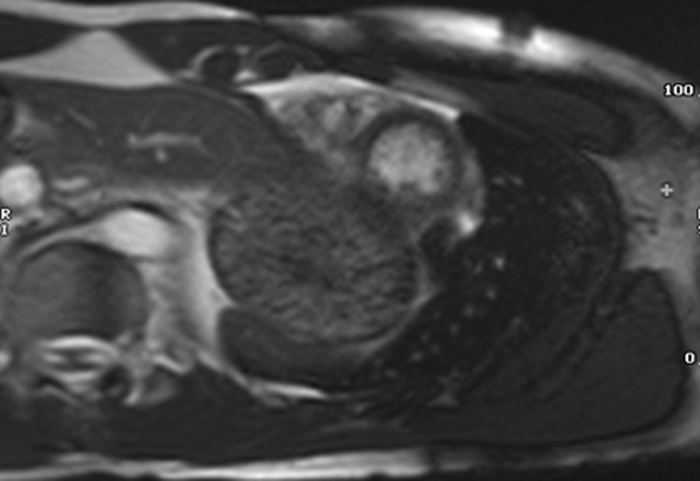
Short-Axis End-Diastolic Cardiac Magnetic Resonance Image Demonstrating Apical and Lateral Hypertrabeculations The noncompacted:compacted myocardial ratio exceeds 3.5 in the apicolateral segment, consistent with left ventricular noncompaction diagnostic criteria.
